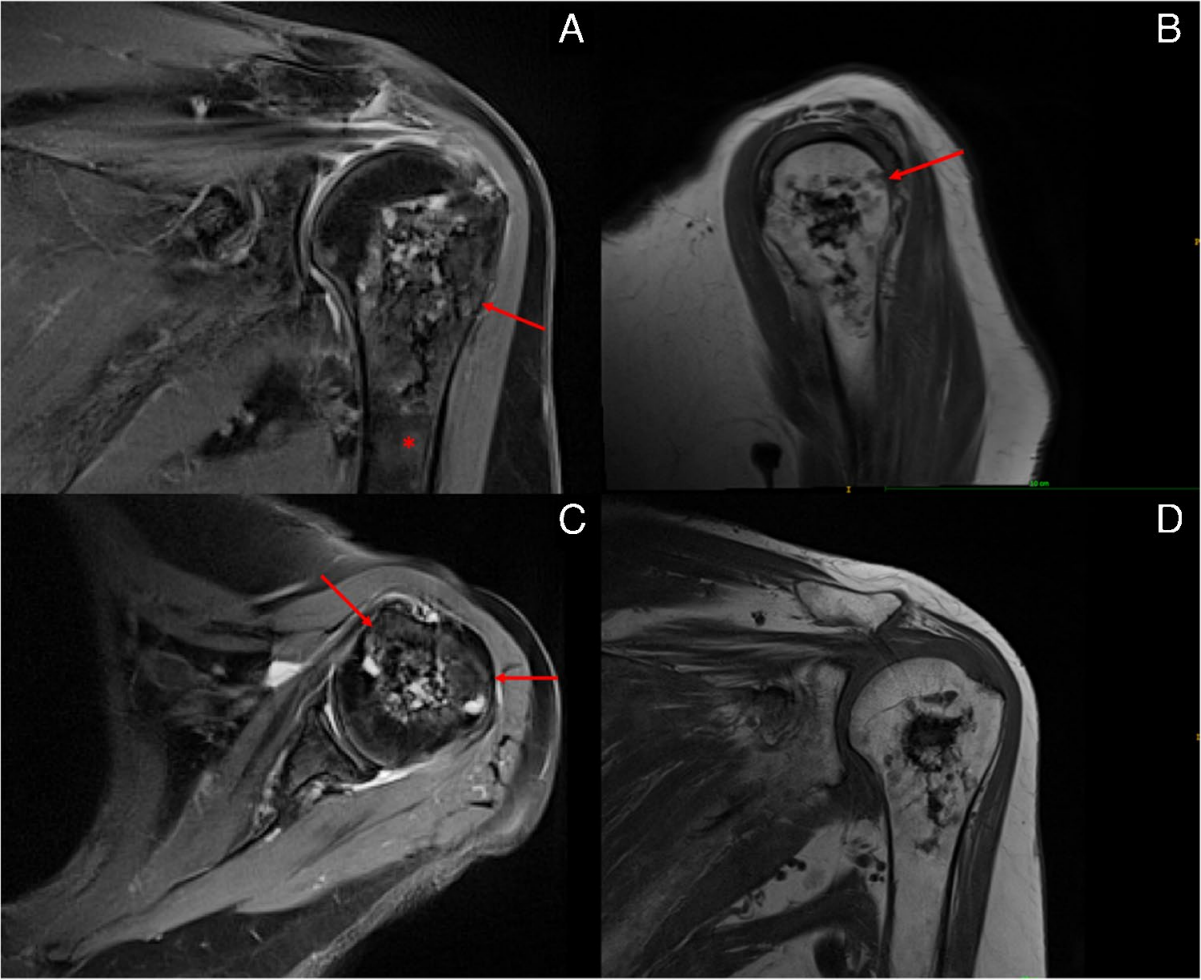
MRI scan of an ACT (maximal diameter of 5.2 cm; deep endosteal scalloping; medullary oedema) of the left humerus: A proton density, blade with fat suppression, coronal; B proton density + t2, fat suppression, dixon turbo spin echo, sagittal; C proton density, blade with fat suppression, transversal; D t1, coronal, turbo spin echo. The arrows point at endosteal scalloping, whereas the asterisk highlights medullary edema
